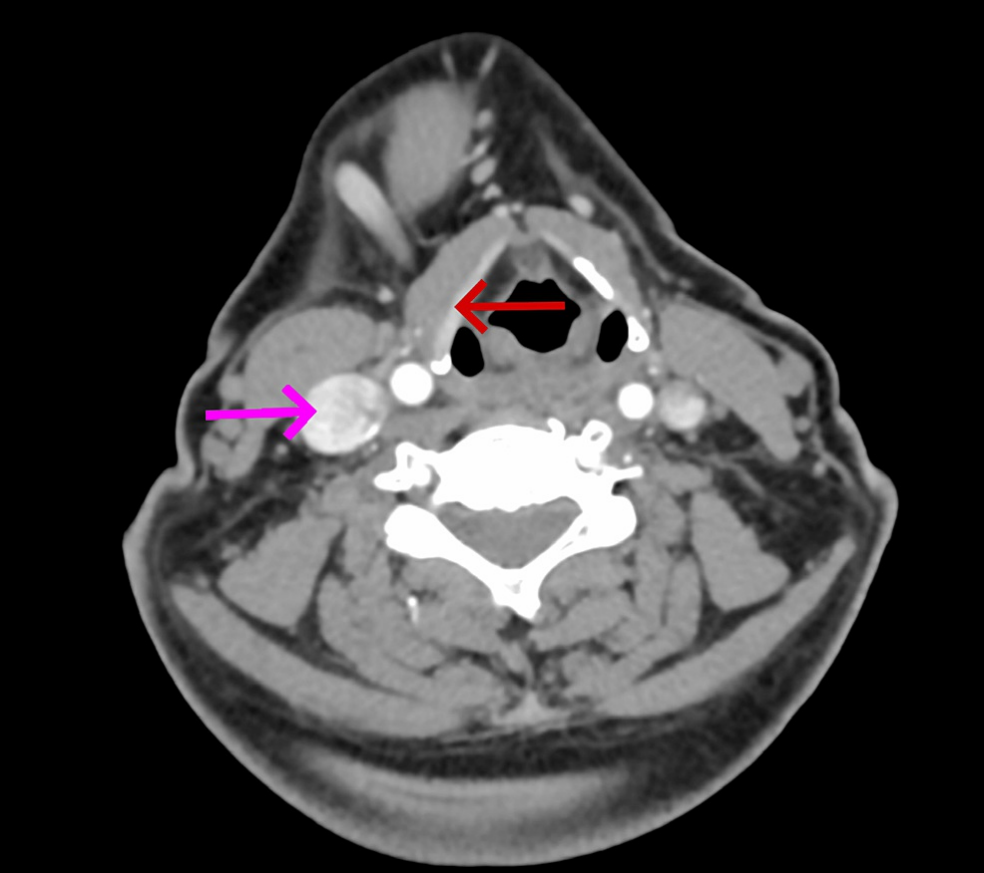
CECT of the neck CECT of the neck shows a well-defined lobulated mass with irregular margins and cystic areas measuring 3.5*3.5*5cm in the right submandibular region extending up to the midline (shown in pink arrow). Also non-visualized thyroid parenchyma suggestive of congenital hypoplastic thyroid (shown in red arrow). CECT - contrast-enhanced computed tomography
